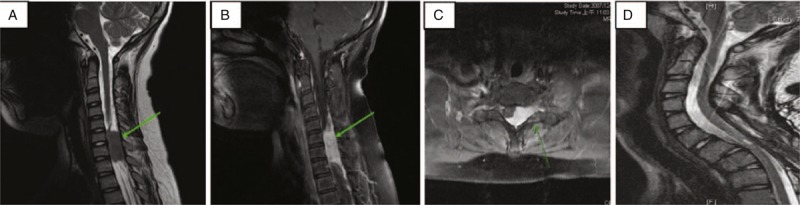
Radiological studies of Case 2. The green arrows mark the position of tumor. (A) Preoperative sagittal T2-weighted imaging of C-spine MRI. (B) Preoperative sagittal Gd-enhanced T1-weighted imaging of C-spine MRI. (C) Preoperative axial Gd-enhanced T1-weighted imaging of C-spine MRI. (D) Sagittal T2-weighted imaging of C-spine MRI at 3 y postsurgery.
